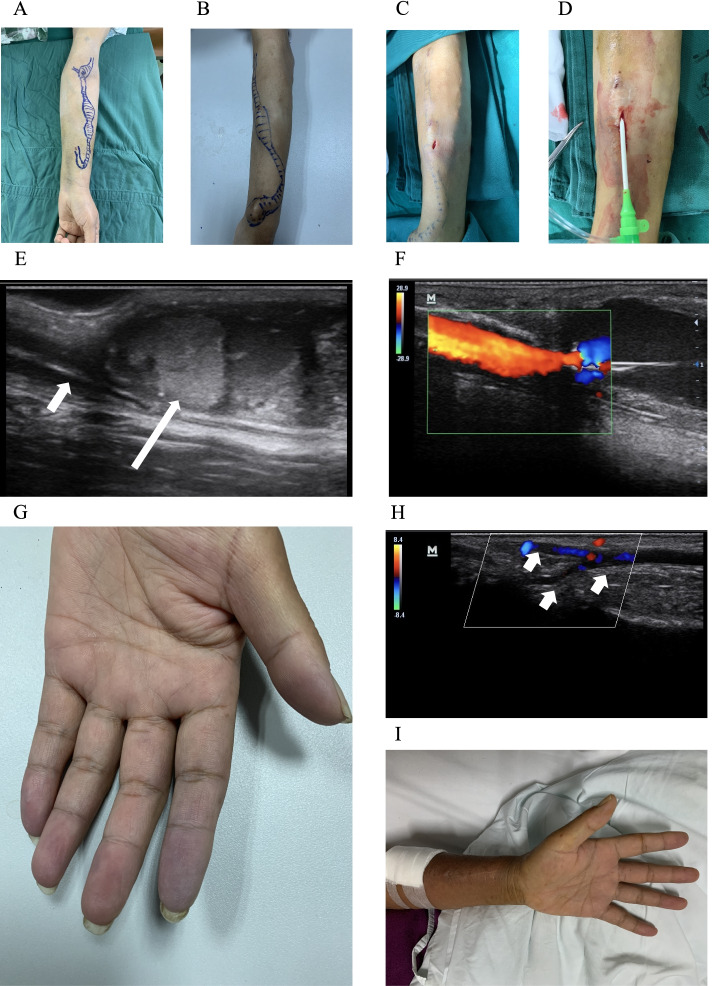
(A and B) Typical thrombosed aneurysmal fistulas. Body surface markings indicate the size of aneurysms and the range of thrombus. (C) The aneurysm wall was exposed using minimal aneurysmotomy. (D) An introducer sheath was inserted antegradely into the incision site under ultrasound guidance. (E) Aneurysm with massive organized thrombi (long white arrow) and proximal stenosis (short white arrow). (F) The color Doppler ultrasound blood flow signal was restored after the organizing thrombus in the aneurysm was removed and the stenosis was fully dilated. (G) The palm of same patient, three digits of the left hand were cyanotic. (H) A preoperative color Doppler ultrasound examination of palmar arch vessels of the patient indicated weak blood flow signal (short white arrow). (I) The cyanosis disappeared after thrombus removal
